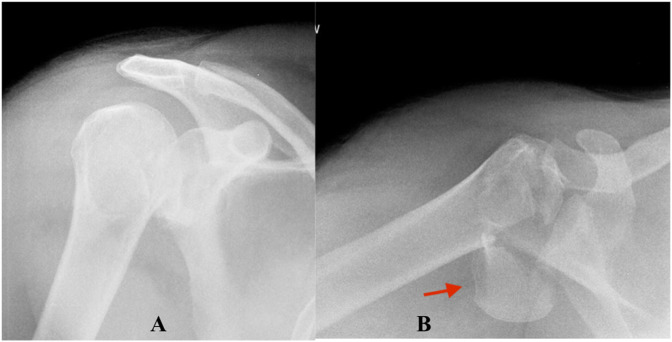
Anteroposterior (A) and lateral (B) radiographs demonstrating a posterior fracture-dislocation of the right shoulder (note red arrow showing a large humeral head fragment).
